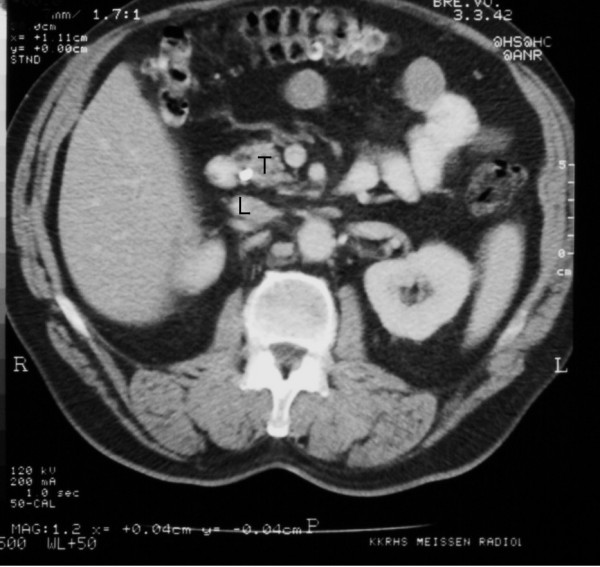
CT after 5 cycles of neoadjuvant chemotherapy with 5-FU. T = tumor; L = residual precaval lymph node.
